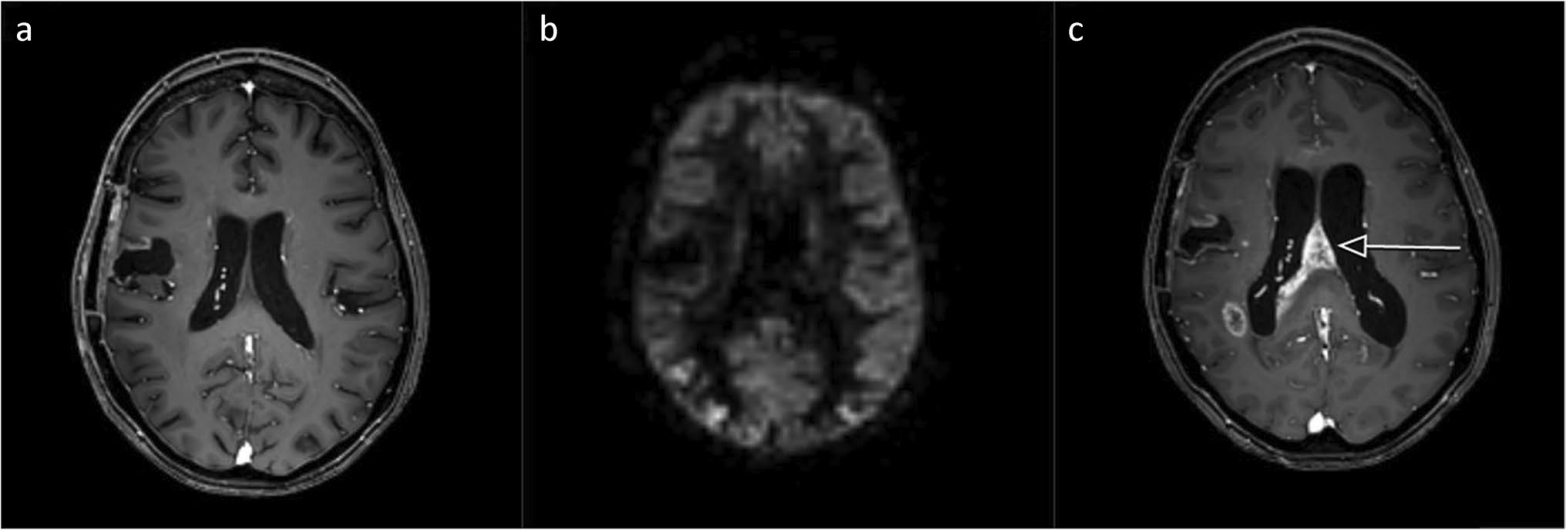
Fifty-five-year-old woman with a diffuse IDH wildtype astrocytoma treated with radiotherapy and temozolomide. False-negative scan; 3.a Index T1-MRI with contrast agent: no contrast enhancement. 3.b Index ASL rated as negative. 3.c Follow-up T1-MRI with contrast agent (203 days after index MRI): new contrast enhancement. All axial planes
